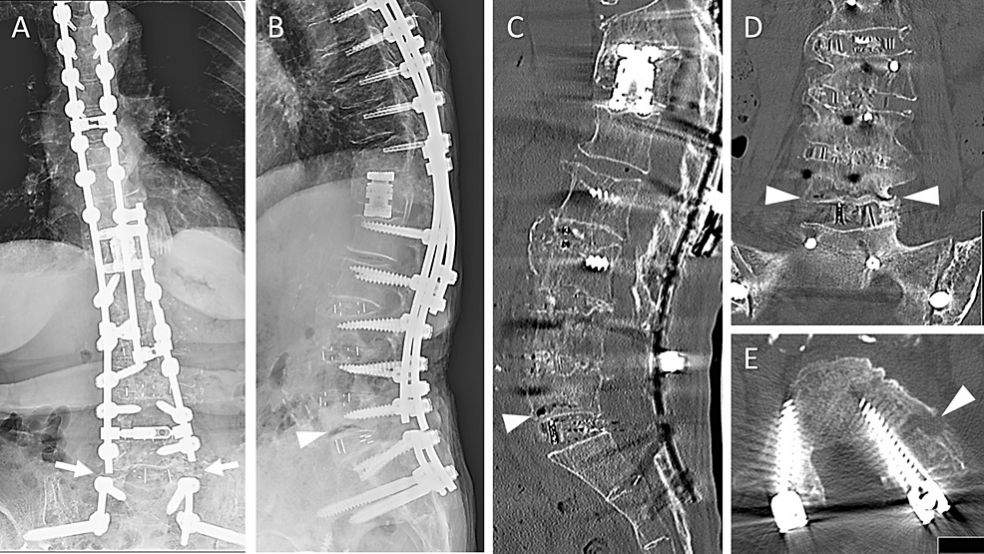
Images of hyperextension vertebral fracture at L5 after bilateral rod fractures. (A, B) Radiographs showed bilateral rod fractures (arrows) and a hyperextension vertebral fracture at L5 (arrowhead). (C, D) CT showed that the vertebral fracture has occurred at the caudal side of the verterbral body (arrowheads). On the other hand, complete interbody fusions at L4-L5 and L5-S1 were detected. (E) CT showed the displaced left pedicle fracture at L5 (arrowhead). CT: computed tomography
